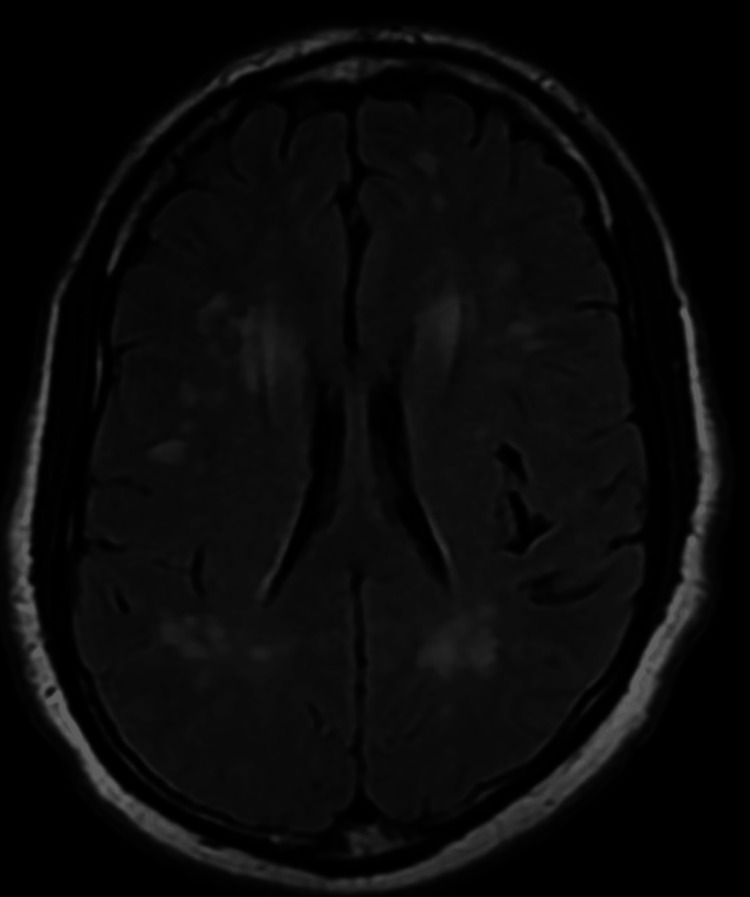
Magnetic resonance imaging fluid-attenuated inversion recovery sequence showing periventricular white matter lesions and deep white matter lesions at the level of the lateral ventricles. They are punctate, bilateral, and diffuse.
